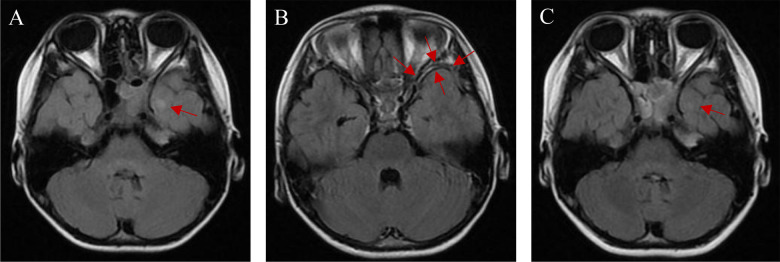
Brain MRI findings in a child with *R. felis* encephalitis. (A) FLAIR imaging on hospital day 3 demonstrating a hyperintense lesion in the left temporal lobe; (B) post-contrast image on hospital day 3 revealing meningeal enhancement in the left temporal lobe; (C) FLAIR imaging on hospital day 20 showing resolution of the lesion.
